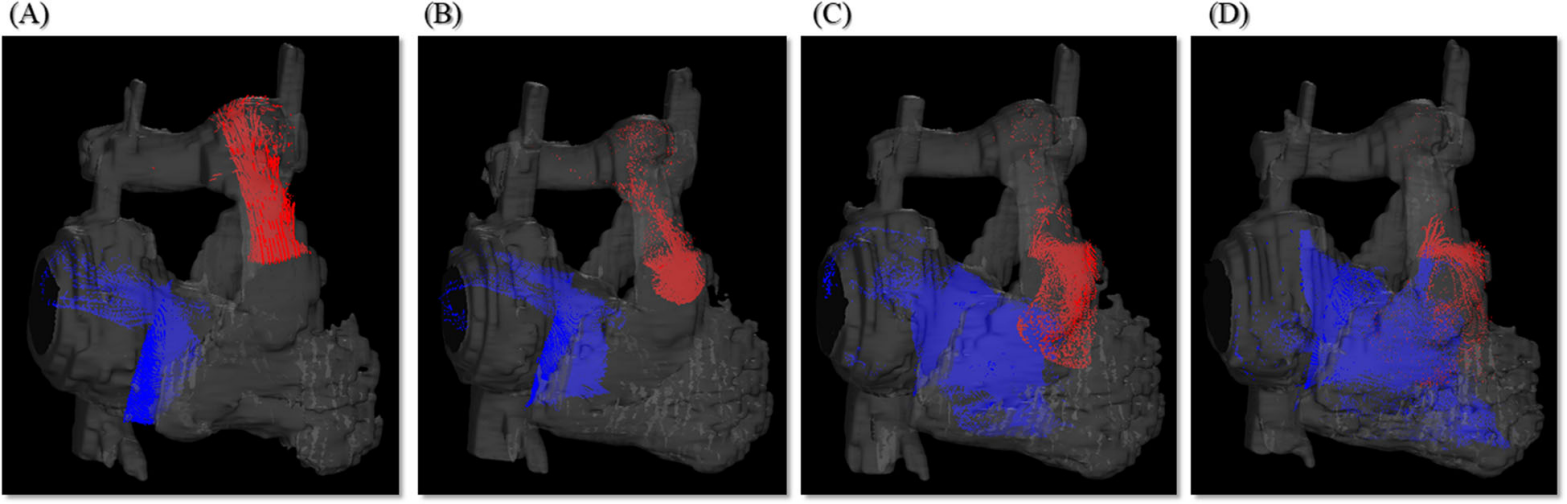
Pulmonary regurgitation and tricuspid regurgitation of three-dimensional path line from four-dimensional flow magnetic resonance imaging. a Early systolic phase, b late systolic phase, c early diastolic phase, and d late diastolic phase
